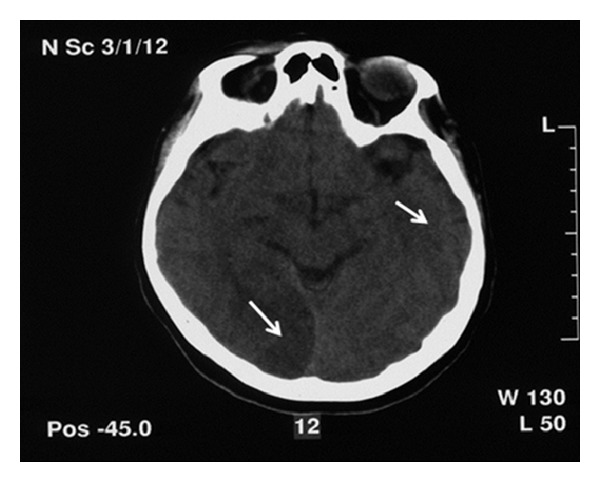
First brain CT scan with multiple infarcts.
